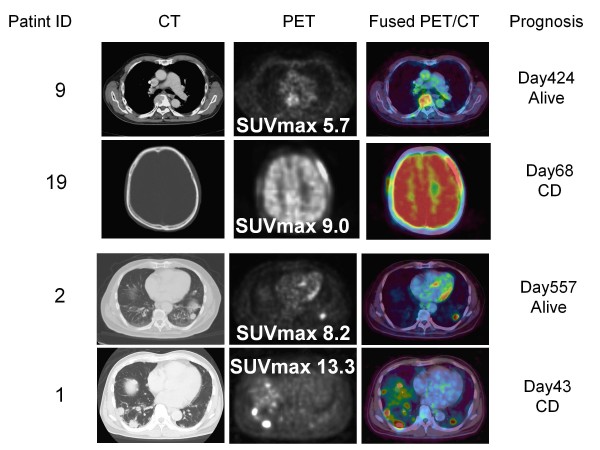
Four Cases with advanced RCC which metastatic sites showed the highest value of SUV among all RCC sites and their prognosis. A cranial bone metastasis showed the highest SUV among all RCC sites in Patient 9. A metastasis in thoracic vertebra did in Patinet 19. Lung metastases did in Patient 1 and Patient 2. The patients with advanced RCCs having high values of SUV max demonstrated poor clinical courses. SUVmax, maximum standardized uptake value; CT, computed tomography; PET, positron emission tomography; Fused PET/CT, fusion of PET and CT.
